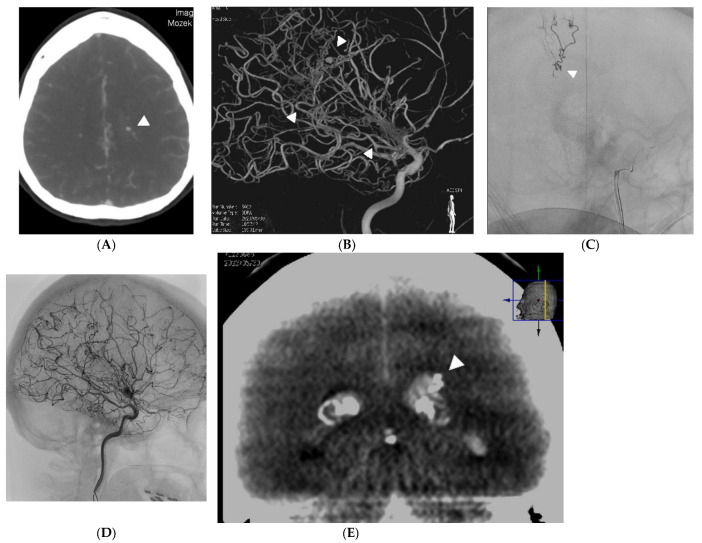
(A) Case 2. CT angiogram showing an aneurysm (arrowhead) in the left lateral ventricle on moyamoya collateral, which was cause of hematocephalus. (B) Case 2. Three dimentional angiogram showing the hypertrophic anterior chorioidal artery (pair of arrows) as collateral bridging the left internal carotid artery intracranially. The contralateral arrow indicates the aneurysm seen on the CT angiogram. (C) Case 2. According to the 3D angiogram, the microcatheter was navigated through the anterior chorioidal artery to the vicinity of the aneurysm. The blood flow in the artery is slowed, so the aneurysm fills only partially and the contrast agent forms a level (arrow). (D) Case 2. Angiogram after acrylic and oily contrast mixture injection shows that the target aneurysm is no longer filling, as well as on control angiography 2 months later. (E) Case 2. Flat panel CT demonstrates embolic mixture penetration into the embolised aneurysm, which was not visible during injection of the embolising agent or on angiography immediately after embolisation. Symmetric calcifications within the lateral ventricles are in the chorioid plexus.
